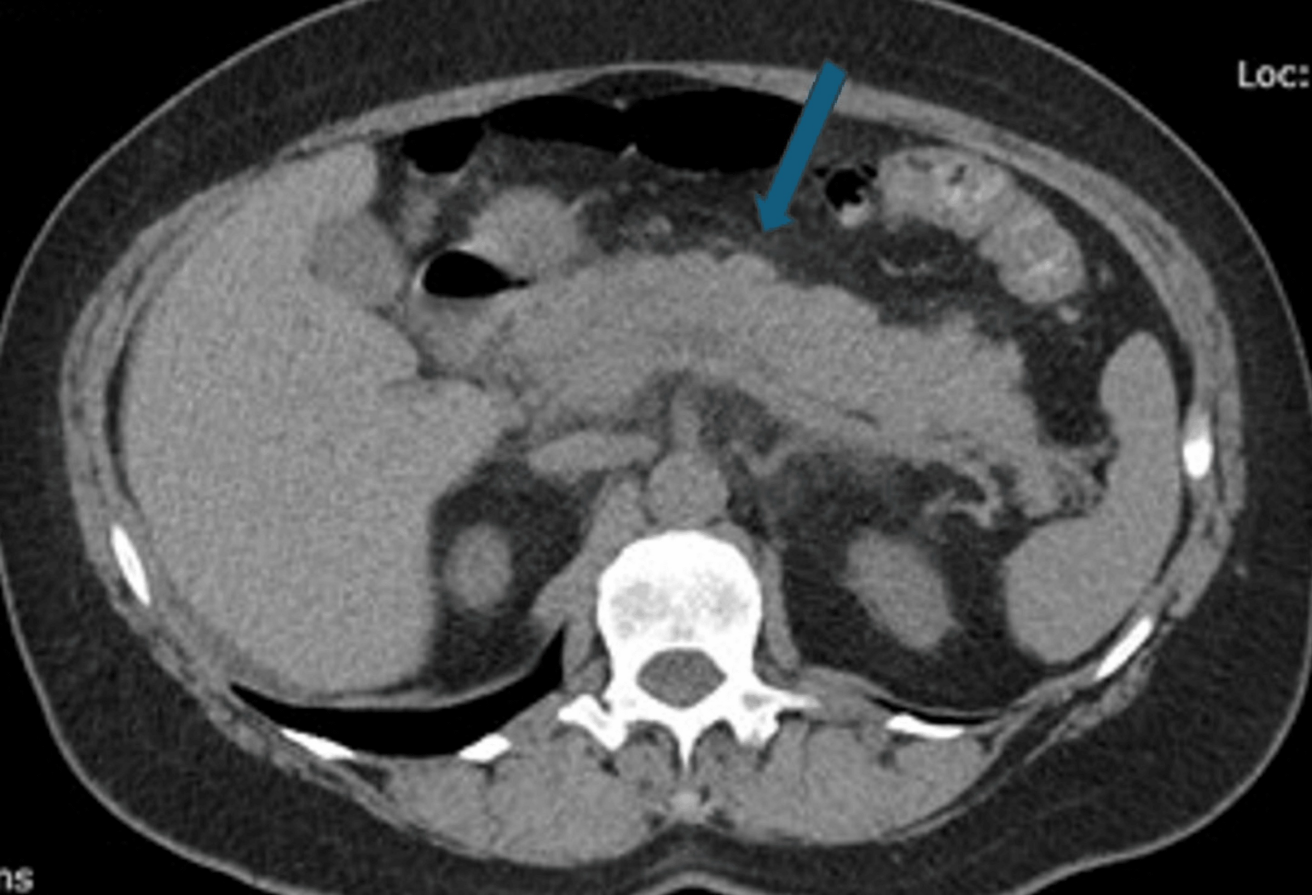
CT abdomen displaying diffuse fat stranding and edema around the pancreatic body, head, and uncinate process (blue arrow).
